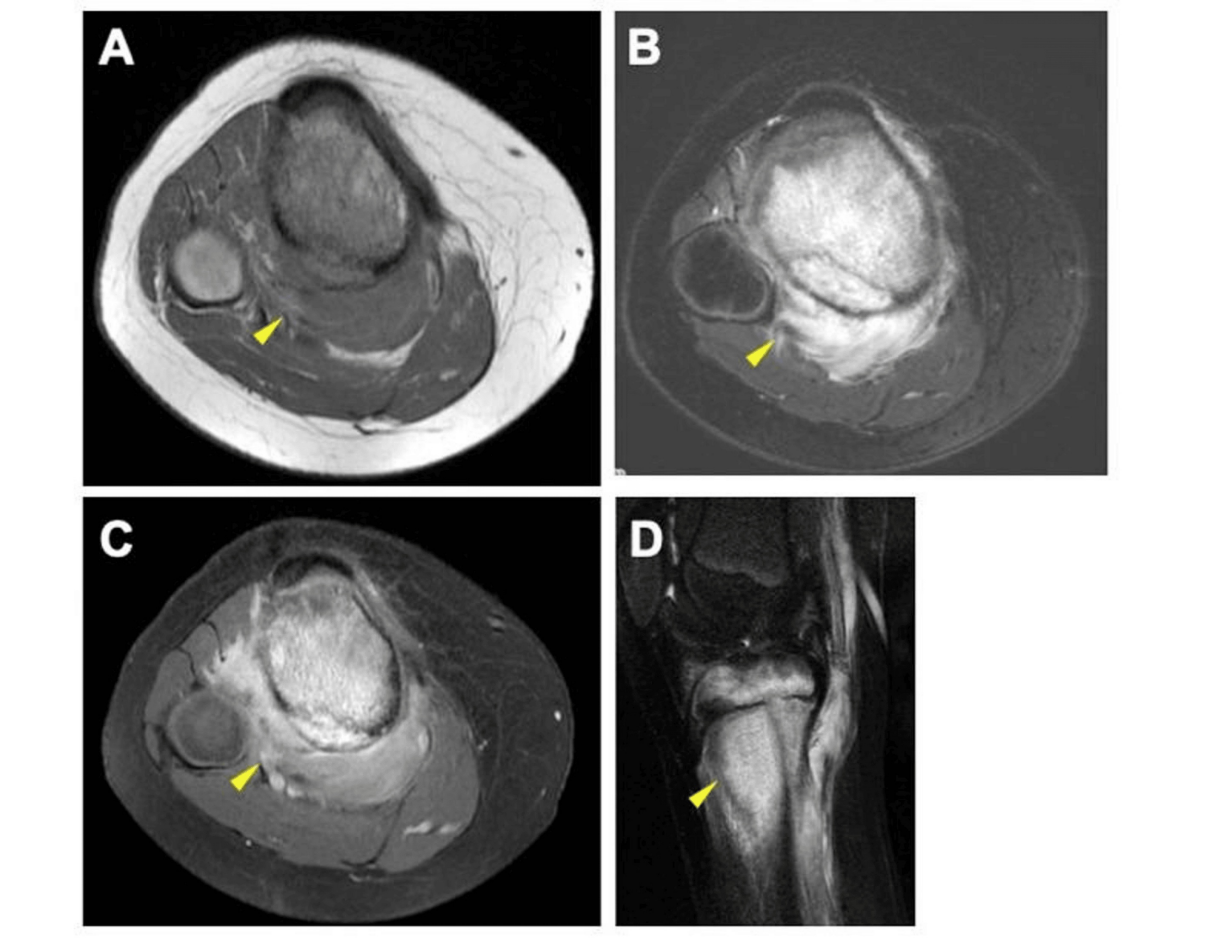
MRI of the proximal tibia. (A) Axial T1-weighted image, (B) axial T2-weighted image, (C) axial gadolinium-enhanced MRI, and (D) sagittal gadolinium-enhanced MRI Magnetic resonance imaging of the proximal tibia metaphysis shows a hypointense mass on a T1-weighted image (A) and a hyperintense mass on a T2-weighted image (B). The mass shows enhancement on a T1-weighted image after administering gadolinium (C) and (D) (yellow arrow).
